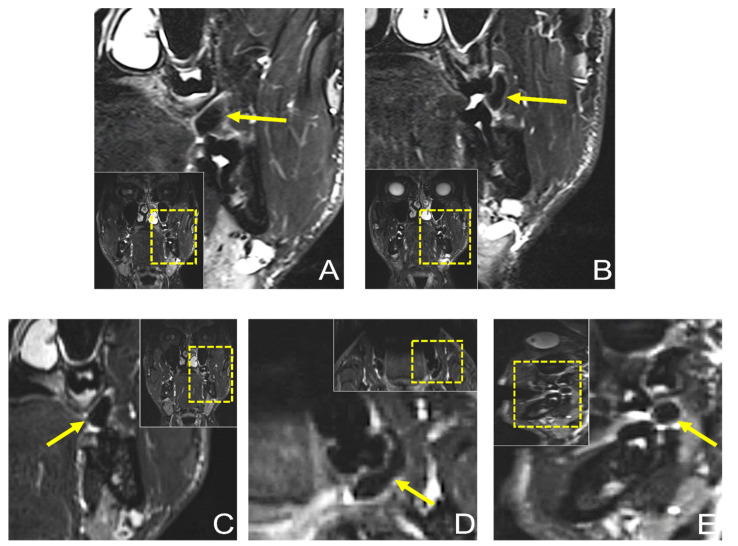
Preoperative dental MRI showed a well-demarcated lesion with a maximum extension of (D) 21.3 × 5.6 mm (axial), (A–C) 13 × 8.6 mm (coronal), and (E) 10.1 × 9.8 mm (sagittal) on a T2 (short-tau inversion recovery) STIR protocol, with homogenous low signal intensity in the central area of the lesion, while the peripheral area showed a high signal intensity. The lesion originated from the planum buccale and did not infiltrate adjacent structures, with (E) a displacement of the second upper molar. For orientation, the dotted rectangles in the corner show the enlarged area.
